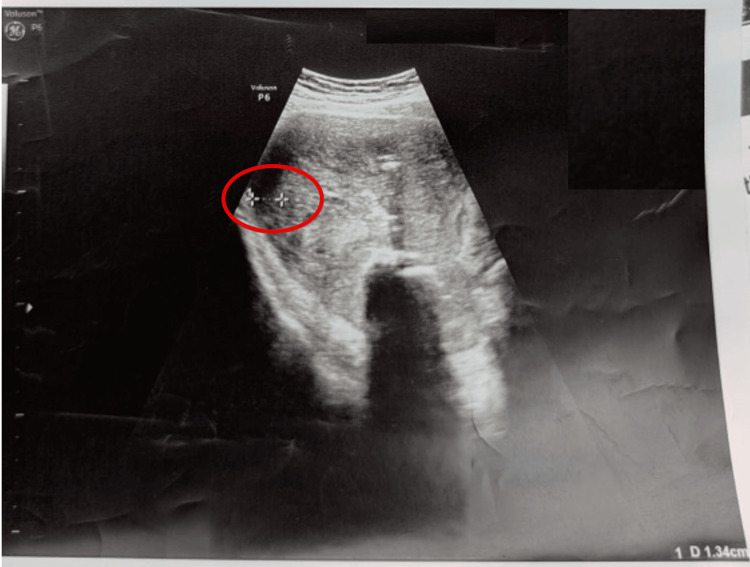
Intraoperative ultrasound shows the thickness of the uterine wall while removing the myoma nascent
